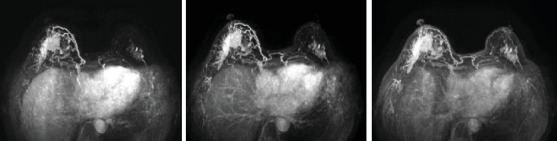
MRI contrast-enhanced dynamic imaging (early, delayed, and late phases) demonstrates diffuse heterogeneous enhancement in the outer quadrant of the right breast, thickening of the right internal mammary artery, and focal heterogeneous enhancement in the outer quadrant of the left breast. All enhancements demonstrate arterial inflow characteristics. BI-RADS V.
